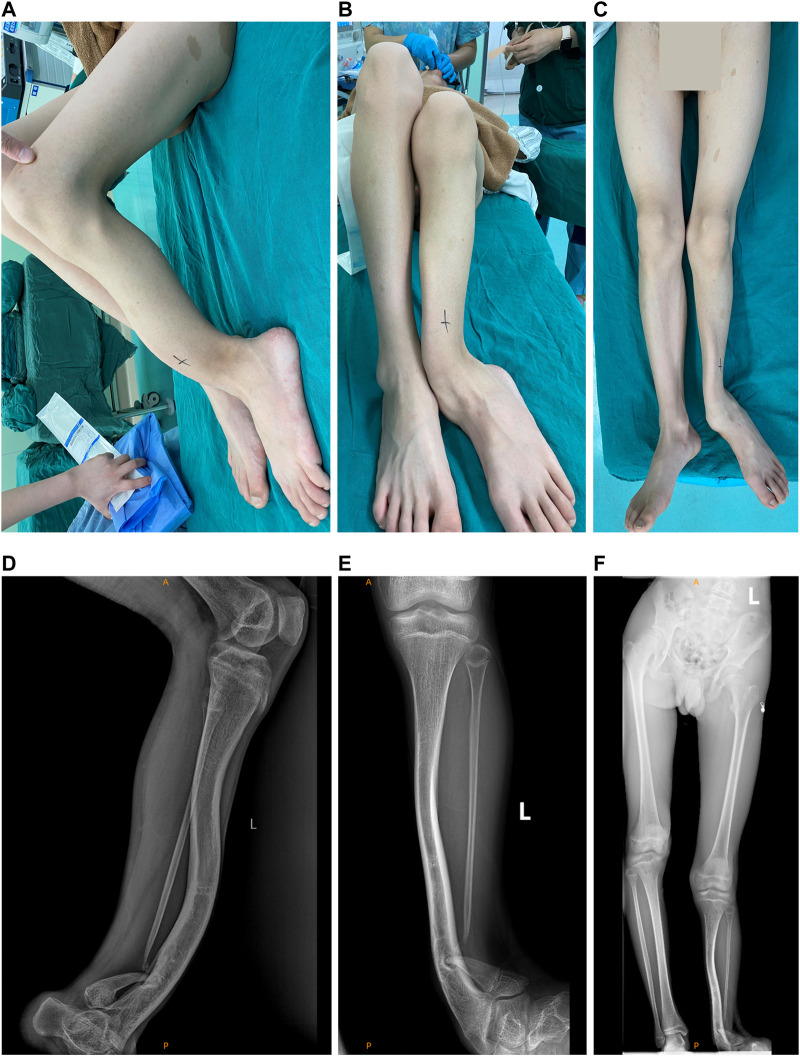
Appearance photos and X-ray images of the left limb of the proband. (A) Lateral photo of the pseudarthrosis of left tibia. (B) Photo of pseudarthrosis of the left tibia and the left ankle valgus. (C) Photo of the unequal lengths of the left and right limb. (D) X-ray image of the pseudarthrosis of left tibia. (E) X-ray image of the pseudarthrosis of the left tibia and the left ankle valgus. (F) X-ray image of the unequal lengths of the left and right limb.
